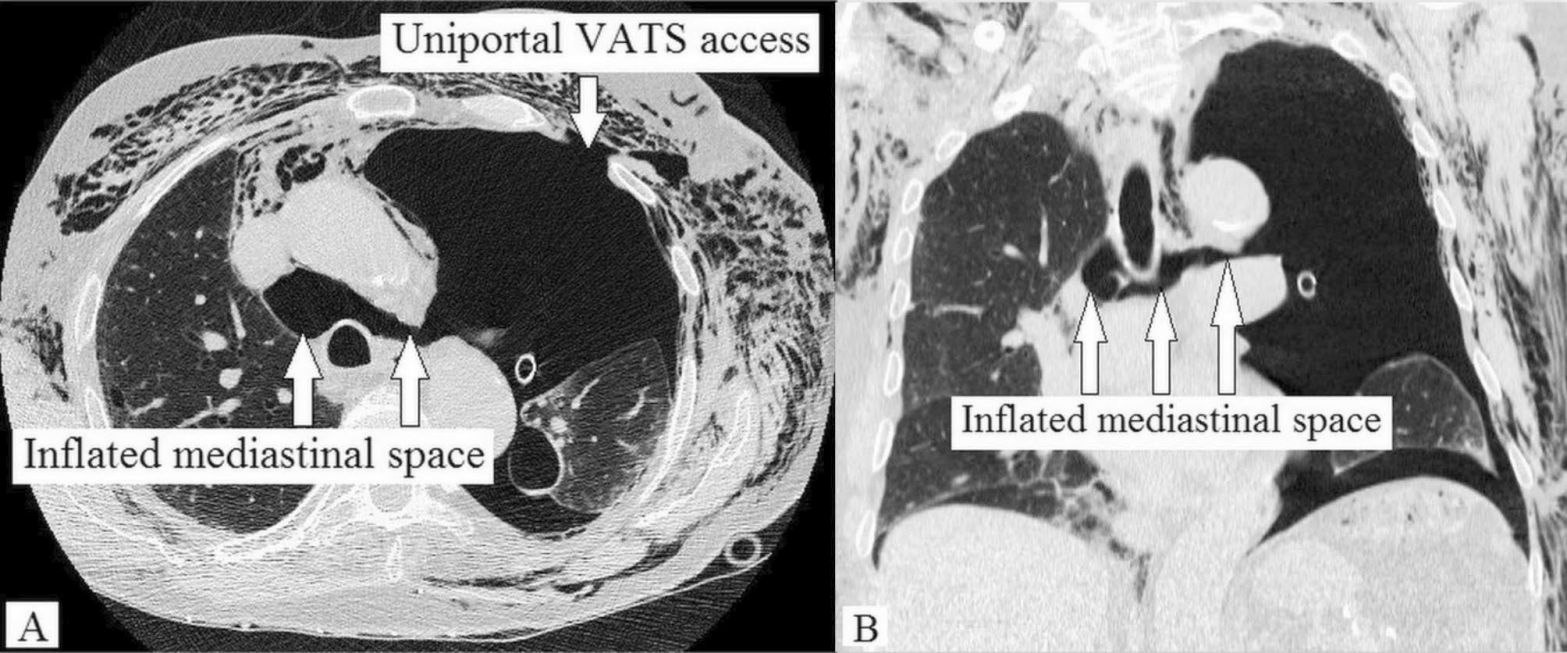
Axial (A) and coronal (B) CT scan showing substantial pneumomediastinum
